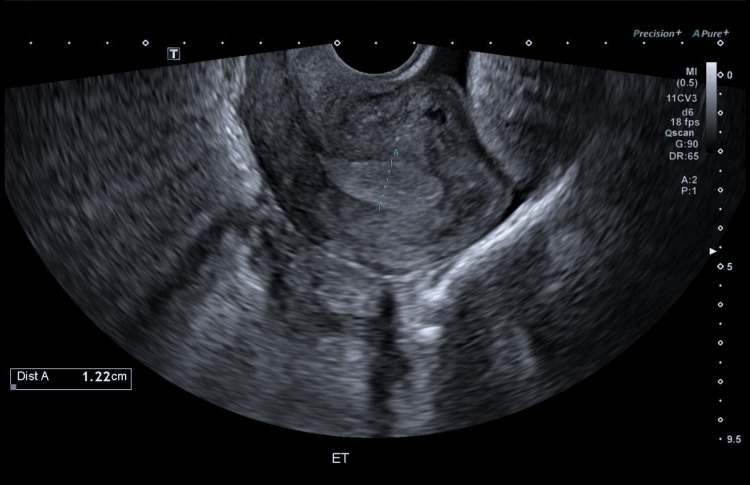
Pelvic ultrasonography Pelvic ultrasonography showing a retroverted uterus and increased endometrial thickness, suggestive of endometrial hyperplasia or endometriosis
